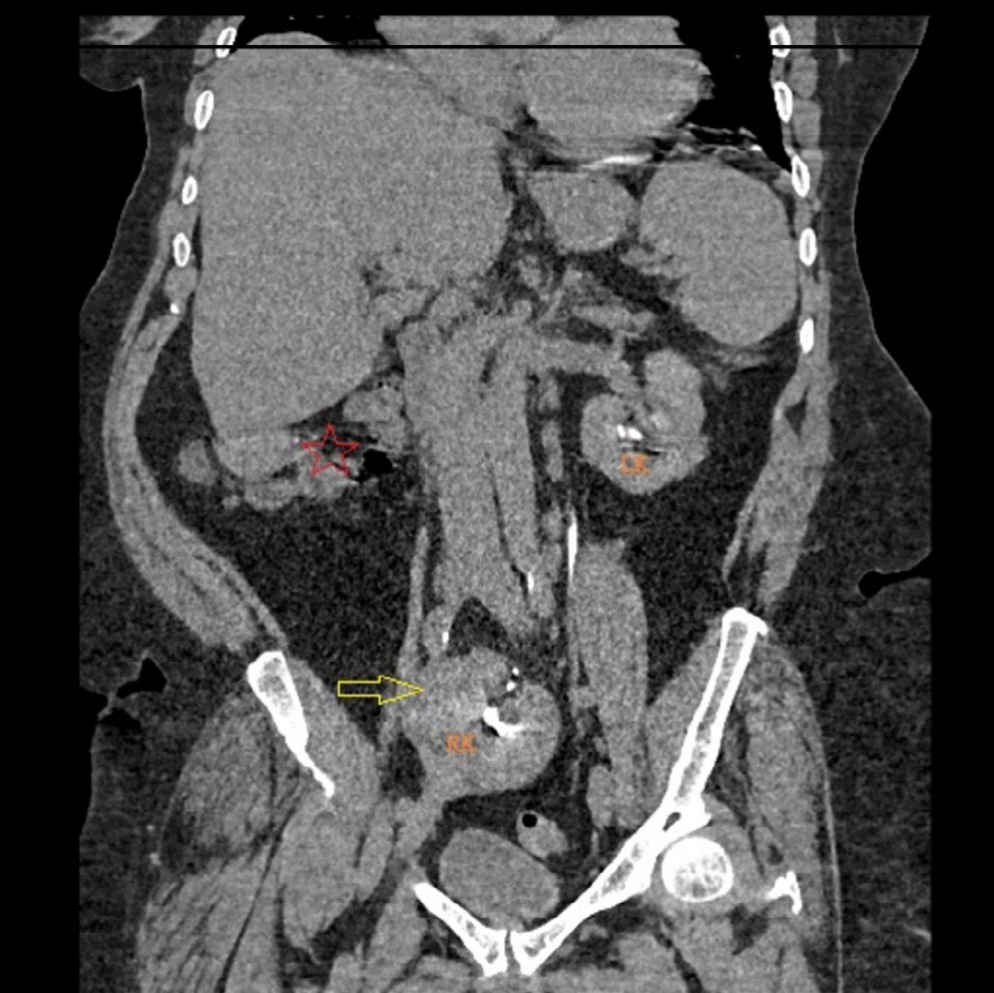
Coronal view, contrast‐enhanced CT scan. This reconstruction demonstrates the ectopic kidney (yellow arrow) in the pelvis and the empty right renal fossa (red star). The left kidney (LK) is in its normal anatomical position.
